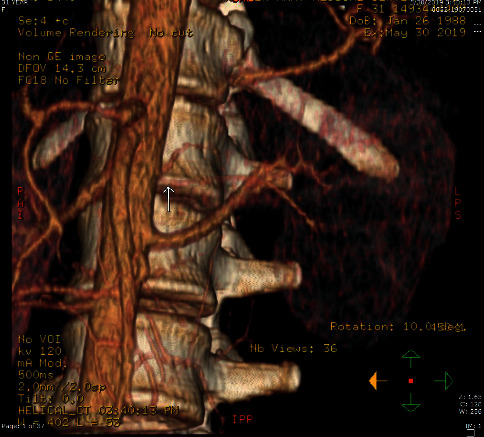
3D image from renal CT with contrast showing the area of ostial stenosis of left accessory renal artery.
